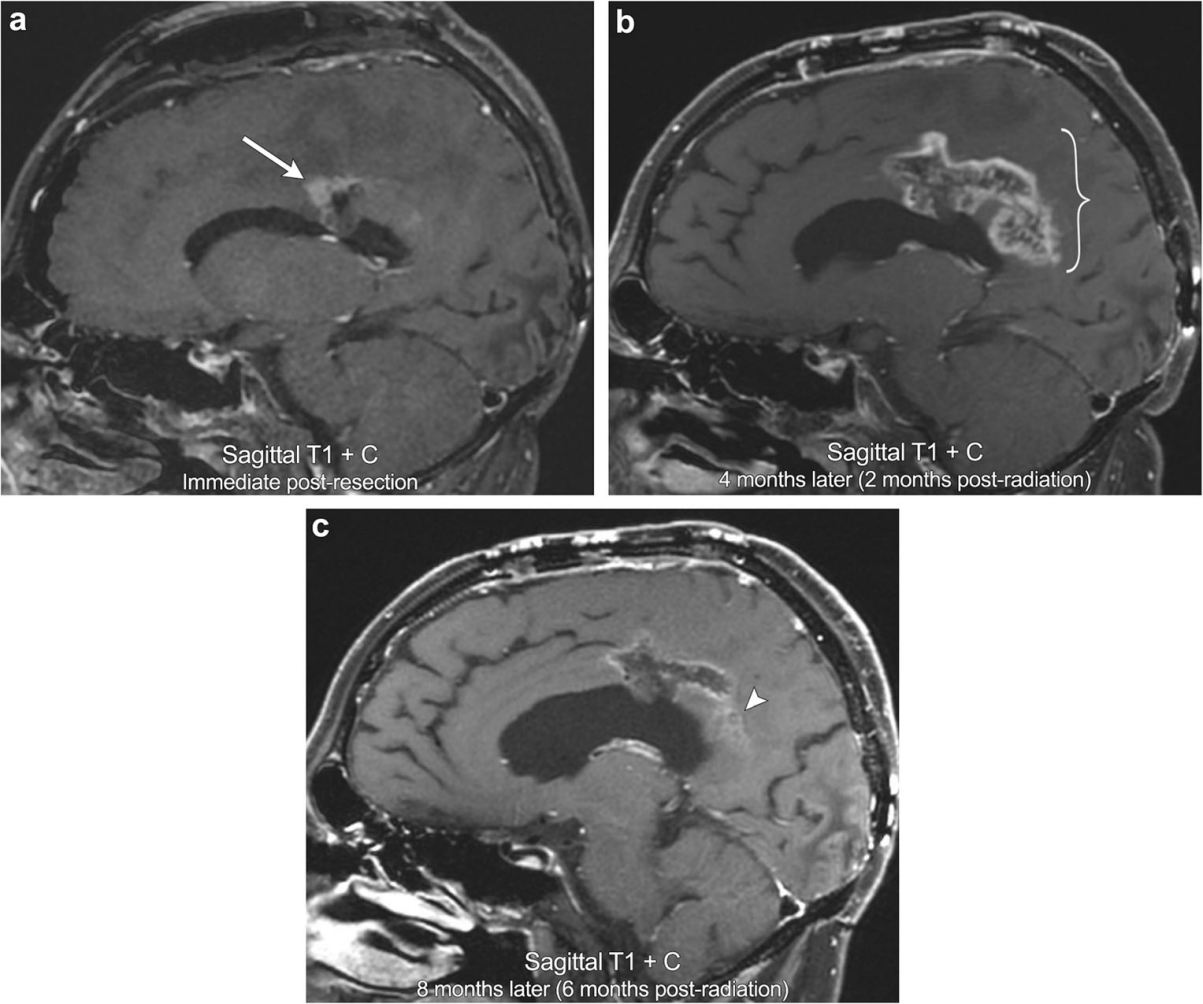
Pseudoprogression. A 46-year-old male with left callosal/pericallosal glioblastoma multiforme. Initial post-resection MRI demonstrates a small area of irregular enhancement along the anterior margin of the resection cavity (arrow). Follow-up imaging obtained 4 months later (2 months after completion of radiation) demonstrates a significant increase in peripheral, now thick, fluffy enhancement along the resection cavity, most marked posteriorly (bracket). Repeat MRI obtained 8 months post resection demonstrates decreased enhancement (arrowhead). No appreciable elevated perfusion or diffusion restriction was present (not shown). The constellation of findings is compatible with pseudoprogression on the 4-month postoperative scan
